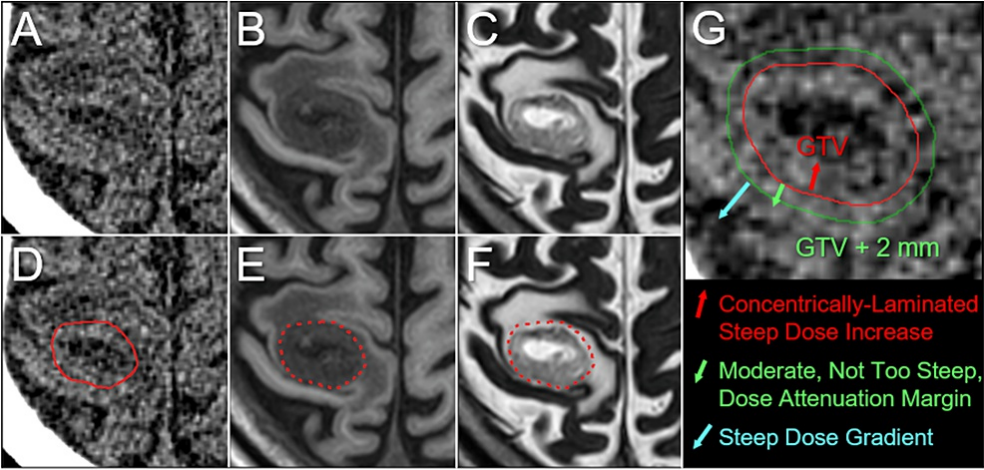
An example (Case 1) of target definition based on non-contrast-enhanced magnetic resonance images and principles of dose distribution design The images show computed tomography (CT) images (A, D, G); T1-weighted images (WI) (B, E); T2-WI (C, F); gross tumor volume (GTV) contour superimposed on the images (D-G) and GTV + 2 mm object (G); our principles of dose distribution design (G); and dose-volume histograms (DVH) (H). (A-F) All images are shown in the same magnification and coordinates under co-registration and fusion. A heterogeneously high-intensity mass lesion on T2-WI with low intensity on T1-WI is distinguishable from the brain parenchyma with or without edema. Although a visible mass on T2-WI is prioritized as a basis for GTV definition, the GTV is finally defined based on a comprehensive comparison of those visible on T2-WI, T1-WI, and CT images. The final GTV contour is slightly larger than the visible mass on T2-WI. (G) The GTV is the most prioritized planning target volume for dose prescription. The GTV + 2 mm object for dose evaluation is generated by adding a uniform 2-mm margin to the GTV.
